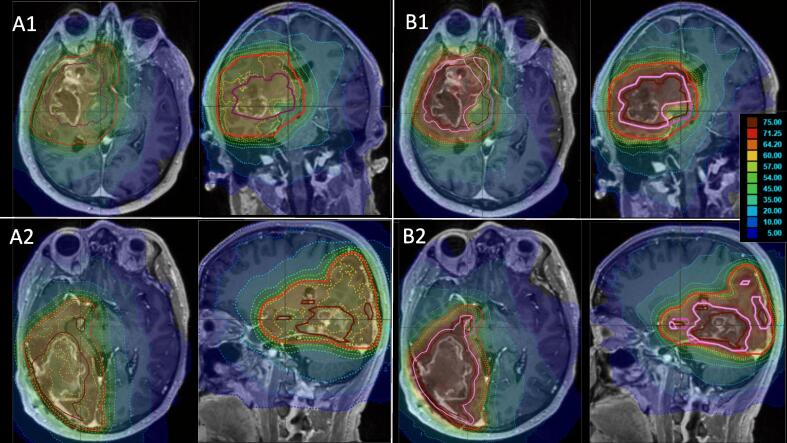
MRI images including dose distribution of two patients, which required a PTVopt due to adjacency to the brainstem and/or optical tract; A1 + 2 are reference plans, B1 + 2 are the experimental plans; A1/B1 is Patient_3; A2/B2 is Patient_7; the red lines is are PTV60 or PTV60ex, dark violet the GTVu, magenta the PTV75, and pink the PTV75opt *(MRI = magnet resonance imaging; GTVu = the union of the gross tumor volume and the biological tumor volume; PTV60, PTV60ex = the planning target volume prescribed with 60 Gy of the reference plan and of the experimental plan; PTV75 = the planning target volume prescribed with 75 Gy of the experimental plan; PTV75opt = the optimized PTV75 for patients adjacent to the brainstem or the optic tract)*.
